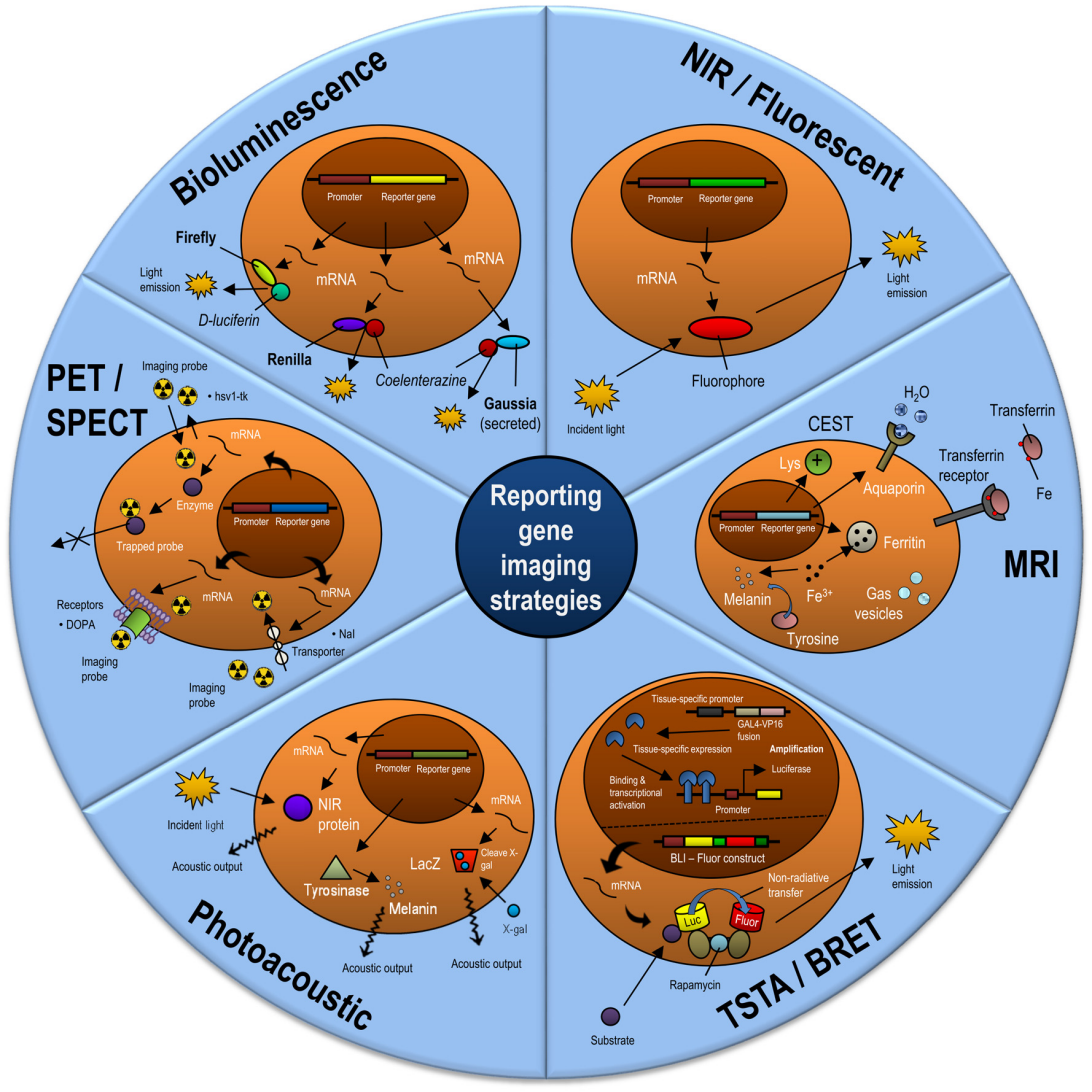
Reporter gene‐based imaging strategies. While NP‐based strategies are primarily focused on cell labeling, many reporter gene strategies have been used to understand underlying biology in addition to imaging cells in vivo. RG strategies for in vivo imaging include near infrared (NIR) and fluorescent RG, MRI RG (ferritin, transferrin, CEST, Tyrosinase, gas vesicles, aquaporin), photoacoustic (Lac Z, Tyrosinase, NIR proteins), PET/SPECT (hsv1tk, dopamine receptor, and sodium iodide transporter), and bioluminescence (Firefly luciferase (Fluc), Renilla luciferase (Rluc), and Gaussia luciferase (Gluc). In each modality, we demonstrate how the RG is expressed and how an imaging signal is generated
